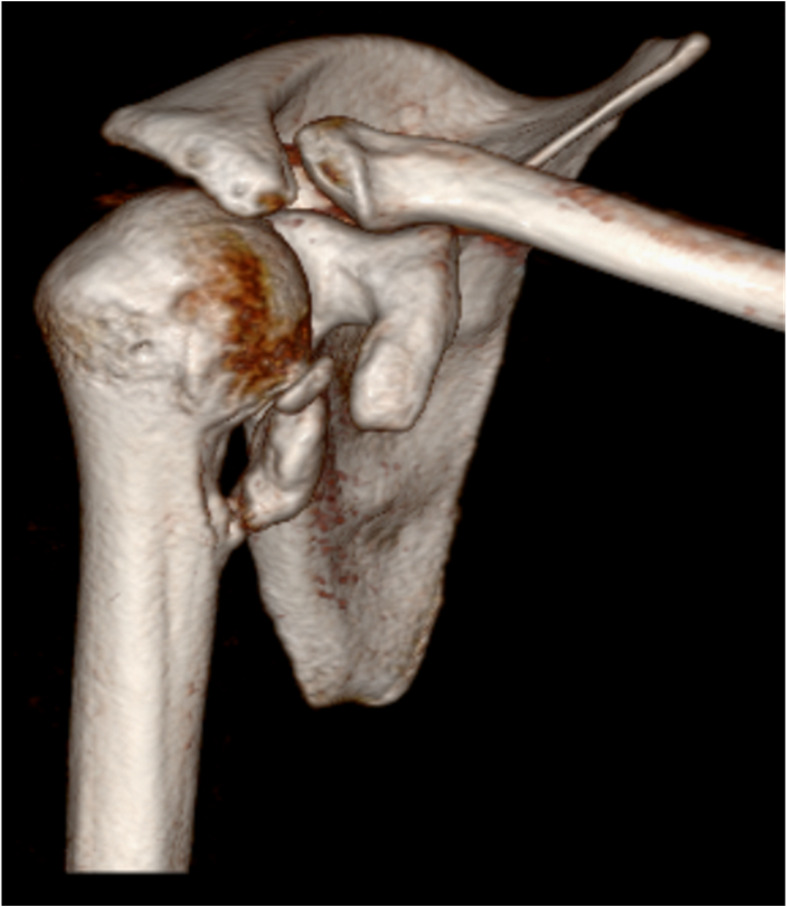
3D reconstruction of the CT-scan
